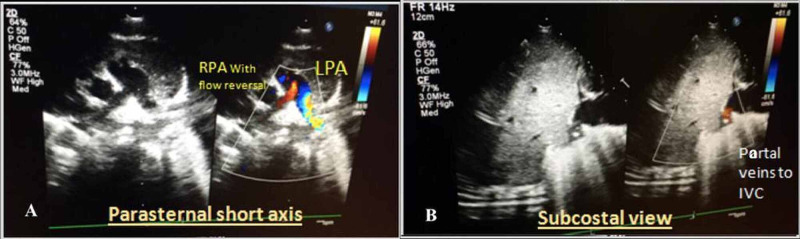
Echocardiography Figure 2A. Right pulmonary artery with flow reversal Figure 2B. Partial vein to inferior vena cava (IVC)
